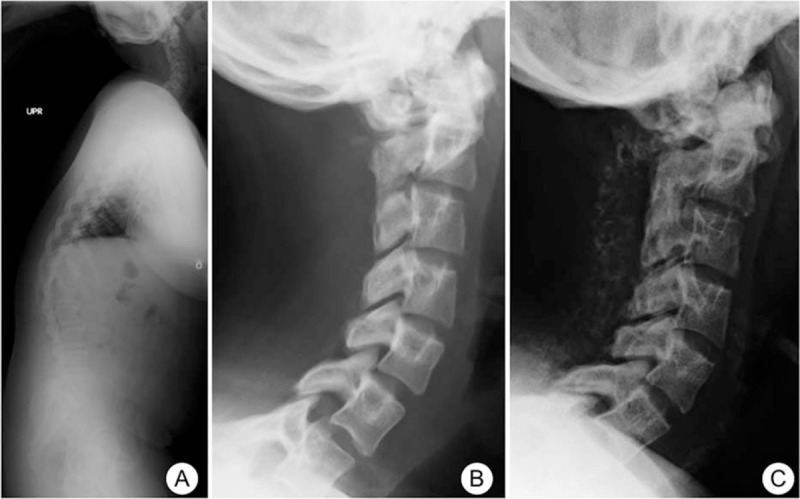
Lateral whole spine radiograph showing congenital thoracolumbar kyphosis and cervical lordosis (A). Cervical lordosis did not change from 1 y (B) to 8 y after surgery (C).
